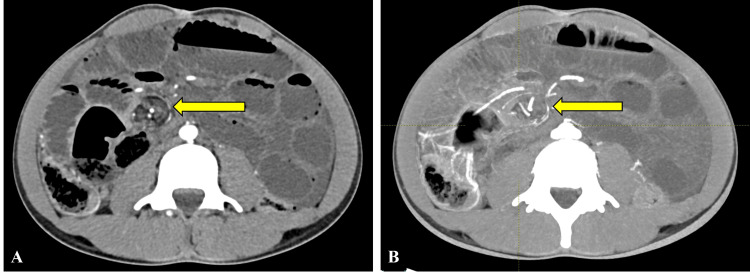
Whirlpool sign Axial sections of the CECT abdomen in thin section (A) and maximum intensity projection (B) show a swirl of mesenteric vessels (yellow arrow) near the fossa of Waldeyer, indicative of the whirlpool sign. CECT: contrast-enhanced computed tomography
